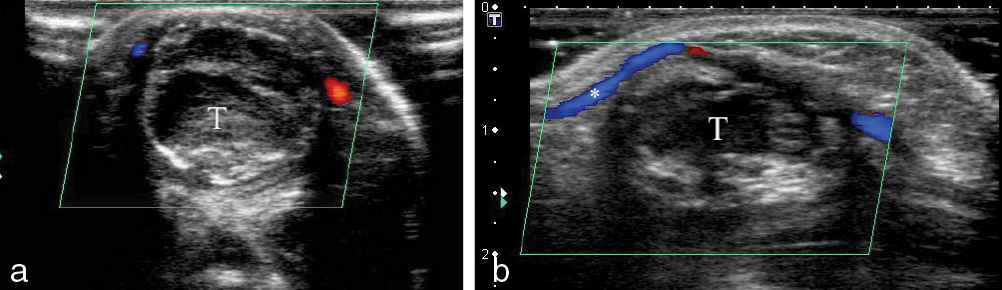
(a) Colour Doppler study on a transverse plane performed after 3 months of expectant management. It shows complete thrombosis (T) and absence of flow within the pseudoaneurysm. (b) Same finding as that in (a) on a longitudinal plane. In this image, good colour Doppler signal can be seen in the brachial artery (*).
